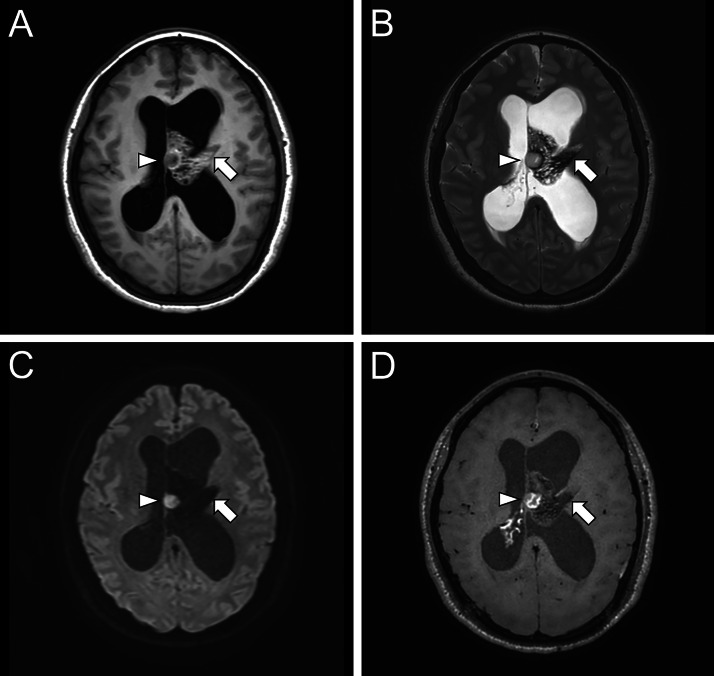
Initial brain MRI. A tumor is seen in the left lateral ventricle, wedge-shaped and continuous with the superior thalamus (arrow), causing hydrocephalus. It appears isointense to the surrounding white matter on T1- weighted imaging (A), and hypointense with internal cysts suggesting a soap-bubble appearance on T2-weighted imaging (B). Diffusion-weighted imaging (b = 1000 s/mm²) (C) and reveals nodular high signal, corresponding to contrast enhancement on postcontrast T1-weighted imaging (D) (arrowhead).
